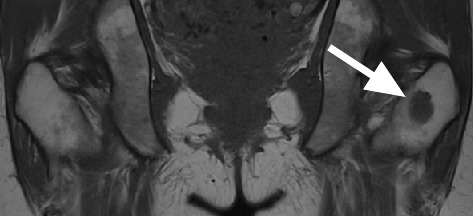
Coronal T1-weighted MRI image demonstrating a well-defined lesion (white arrow) in the upper left femur.
